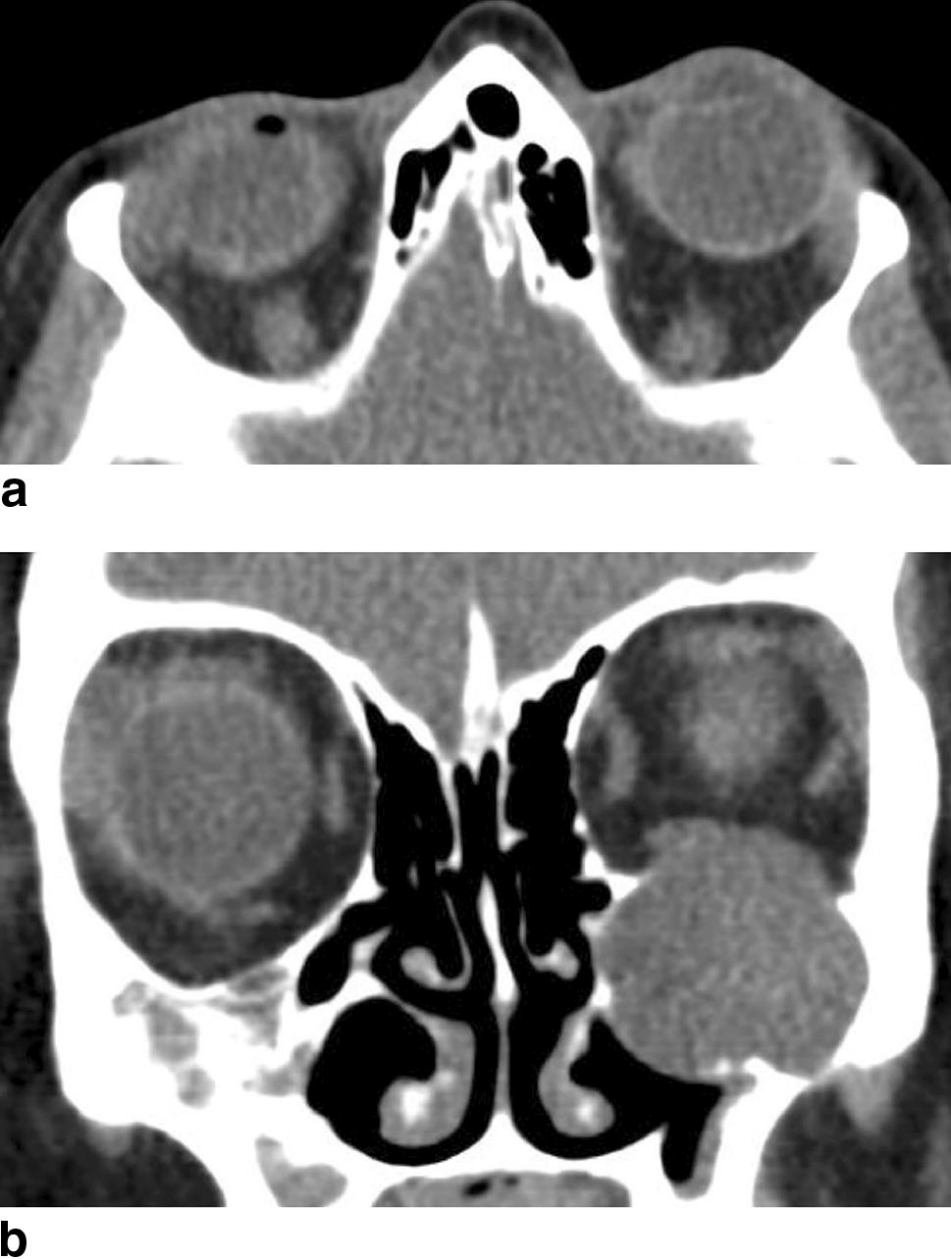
(a,b) Possible challenges in axial CT measurement of proptosis: Bias caused by no section available bisecting both lenses due to apparent vertical displacement of the left globe resulting from a mucocele of the maxillary sinus [(a) axial and (b) coronal CT with contrast agent, soft tissue window].
